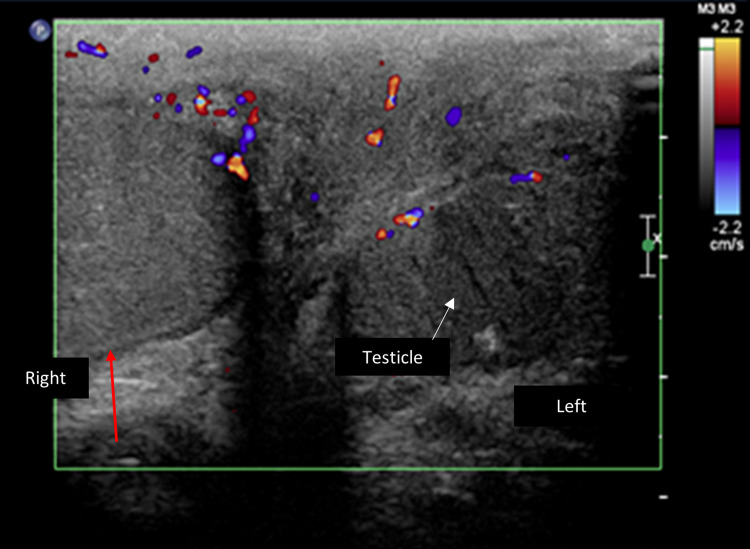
Scrotal ultrasound ruled out underlying collections. The image is showing subcutaneous thickening and increased subcutaneous vascularity suggestive of scrotal cellulitis (red arrow: subcutaneous thickening; white arrow: left testicle).
